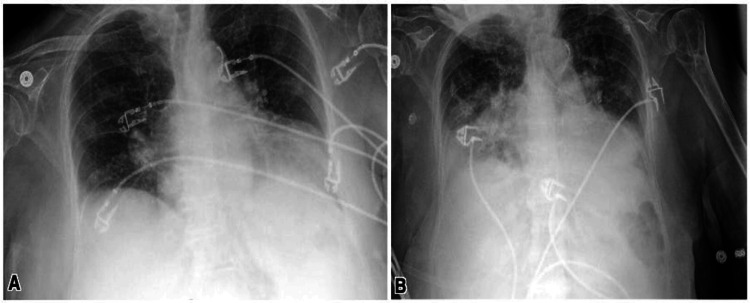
Chest X-ray on admission showed diminished lung volumes, clear lungs, and cardiomegaly. The aorta is noted to be tortuous and calcified (A). Chest X-ray on hospital day 3: new right lower lobe alveolar opacity consistent with pneumonia (B).
